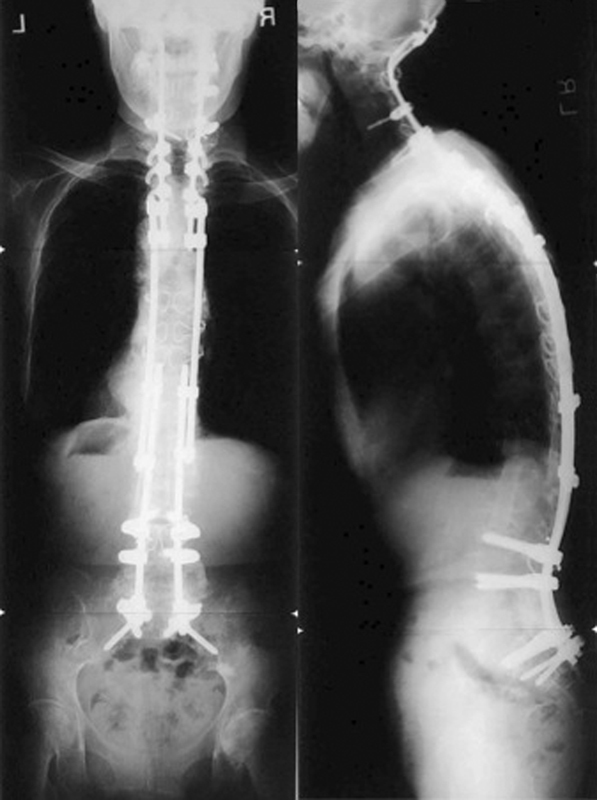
Anteroposterior and lateral radiography of the whole spine after occipitosacral fusion. Anterior fibula strut bone graft was also done at T10–T12 and T12–L3.
